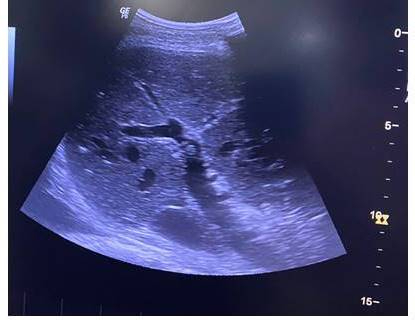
Hepatic arteriovenous malformations seen on upper abdominal ultrasonography.
